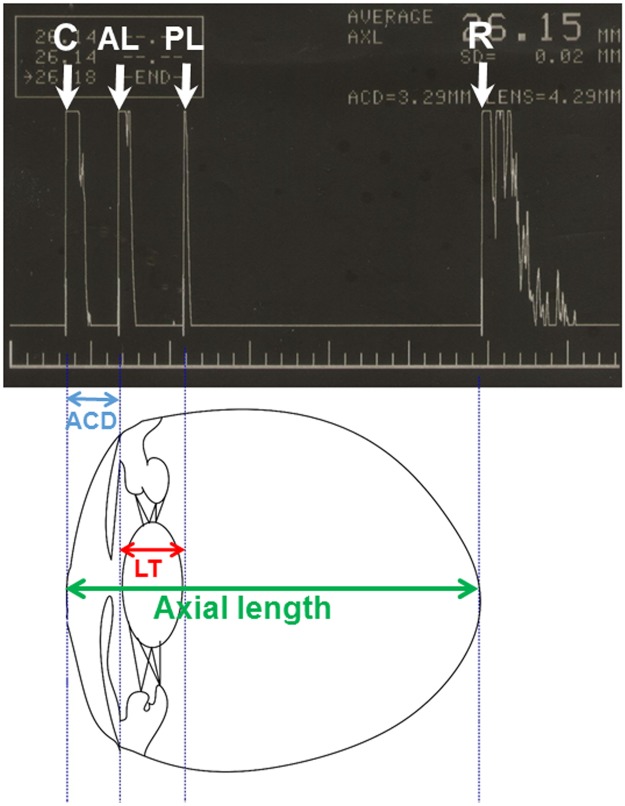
A-scan ultrasonography image along the optical axis of an eye. Peaks correspond to cornea (C), anterior lens (AL) surface, posterior lens (PL) surface, and retina (R). Anterior chamber depth (ACD), lens thickness (LT) and axial length can be derived from A-scan ultrasonography.
